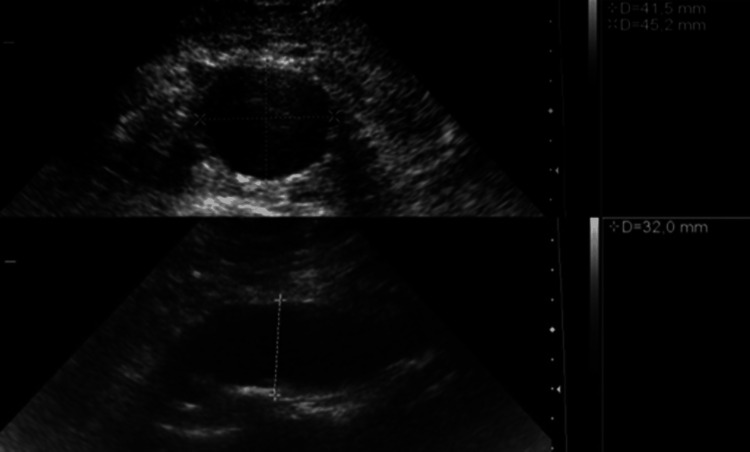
US Measurements of AD in Transverse (Top) and Longitudinal (Bottom) Planes. AD, aortic diameter; US, ultrasound
